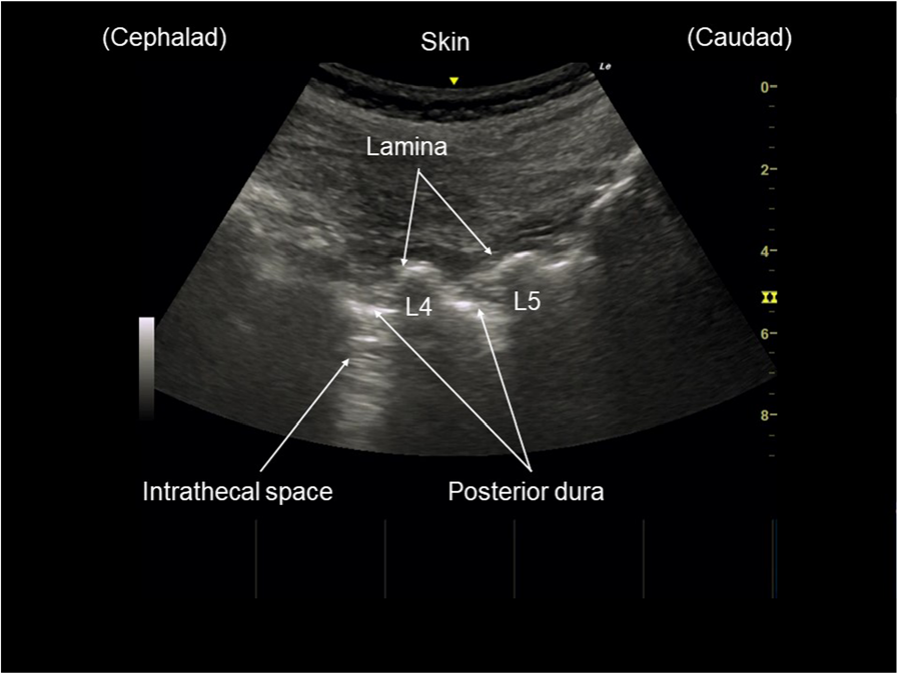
An ultrasound image obtained immediately before puncture when the transducer was placed in a paramedian sagittal oblique plane
